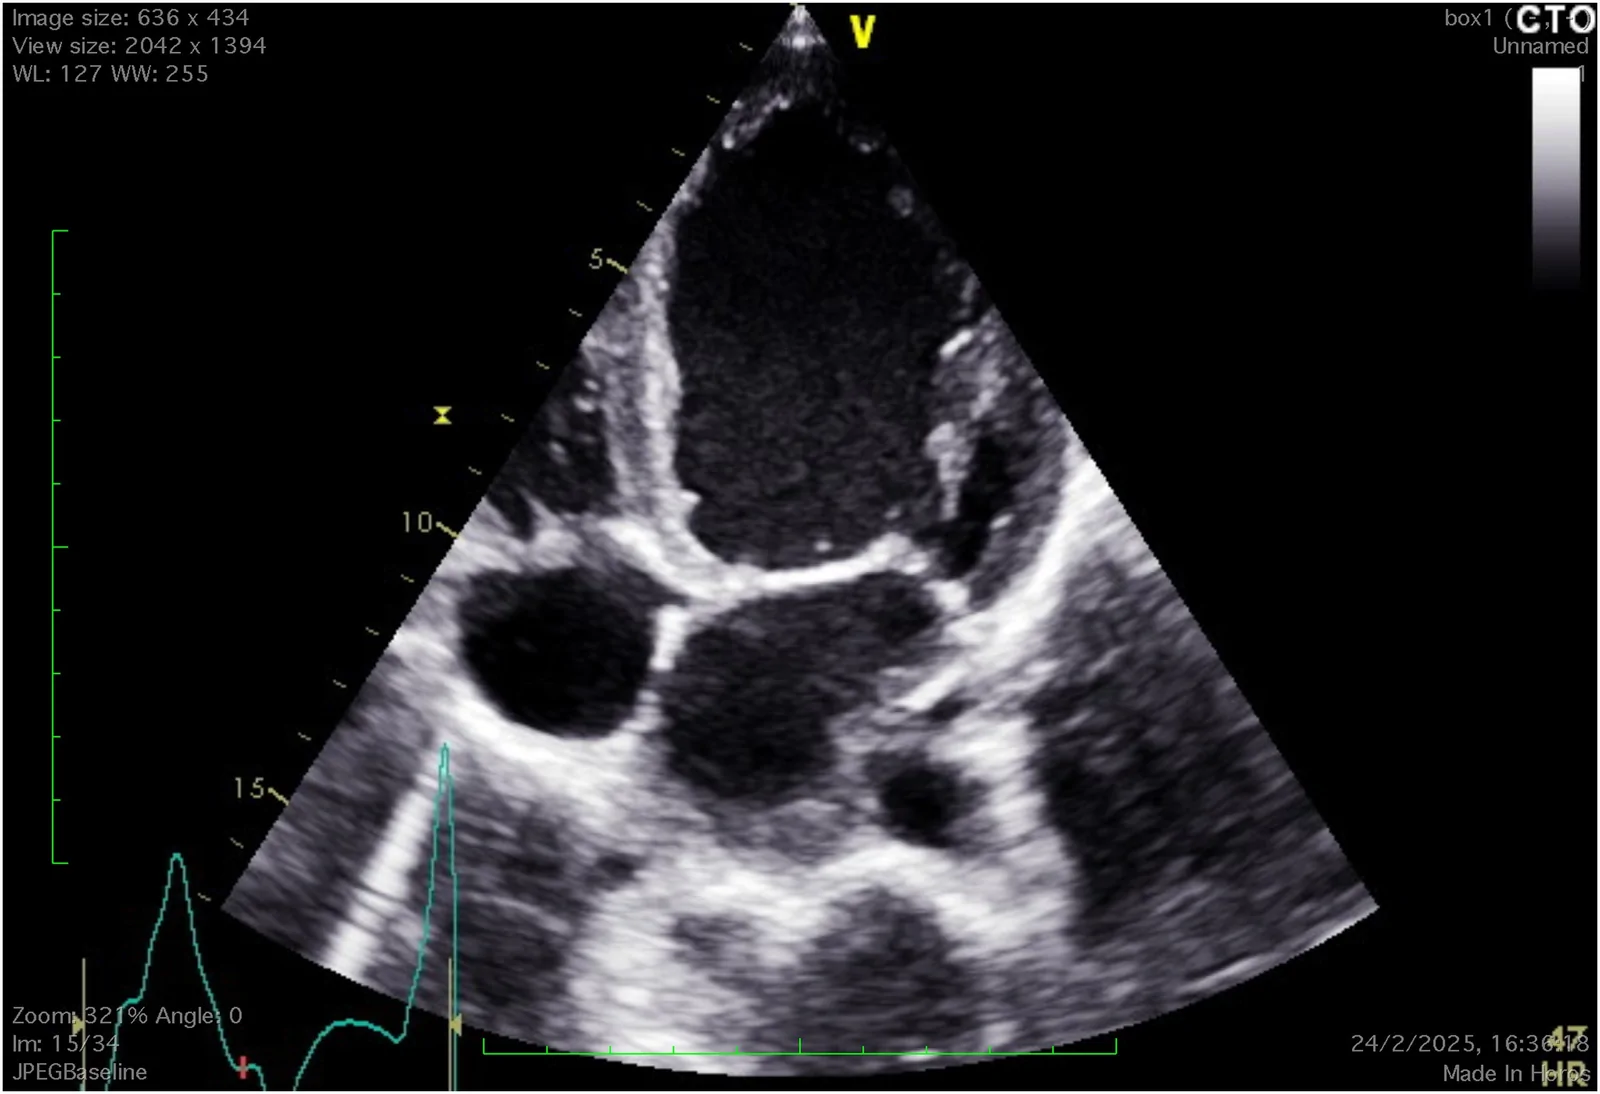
Initial transthoracic echocardiography. Apical four-chamber transthoracic echocardiographic view obtained at presentation showing marked left ventricular dilatation in the context of severe global systolic dysfunction. Left ventricular ejection fraction was estimated at 21% by the Simpson biplane method. No regional wall-motion abnormality was identified, and right ventricular systolic function was preserved.
